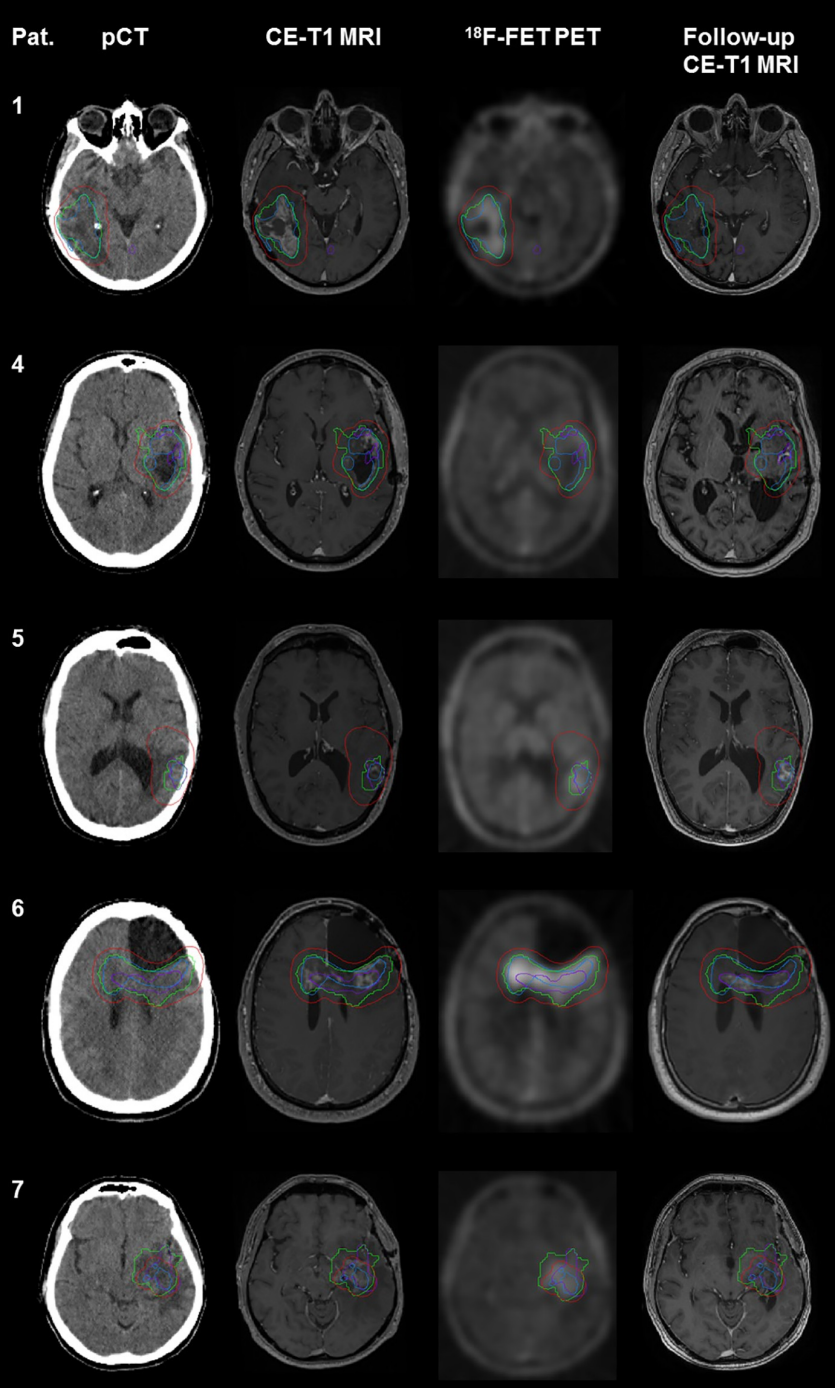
Treatment plan and pattern of recurrence. Original MRI based GTVs in blue, _PET-MR_GTVs in green, CTVs resembling original PTVs minus 3 mm in red and recurrent tumour volumes in follow-up MRI in purple for all patients with available follow-up MRI at recurrence. In patients 1, 4 and 7 no PET information was contained in the original target volumes.
